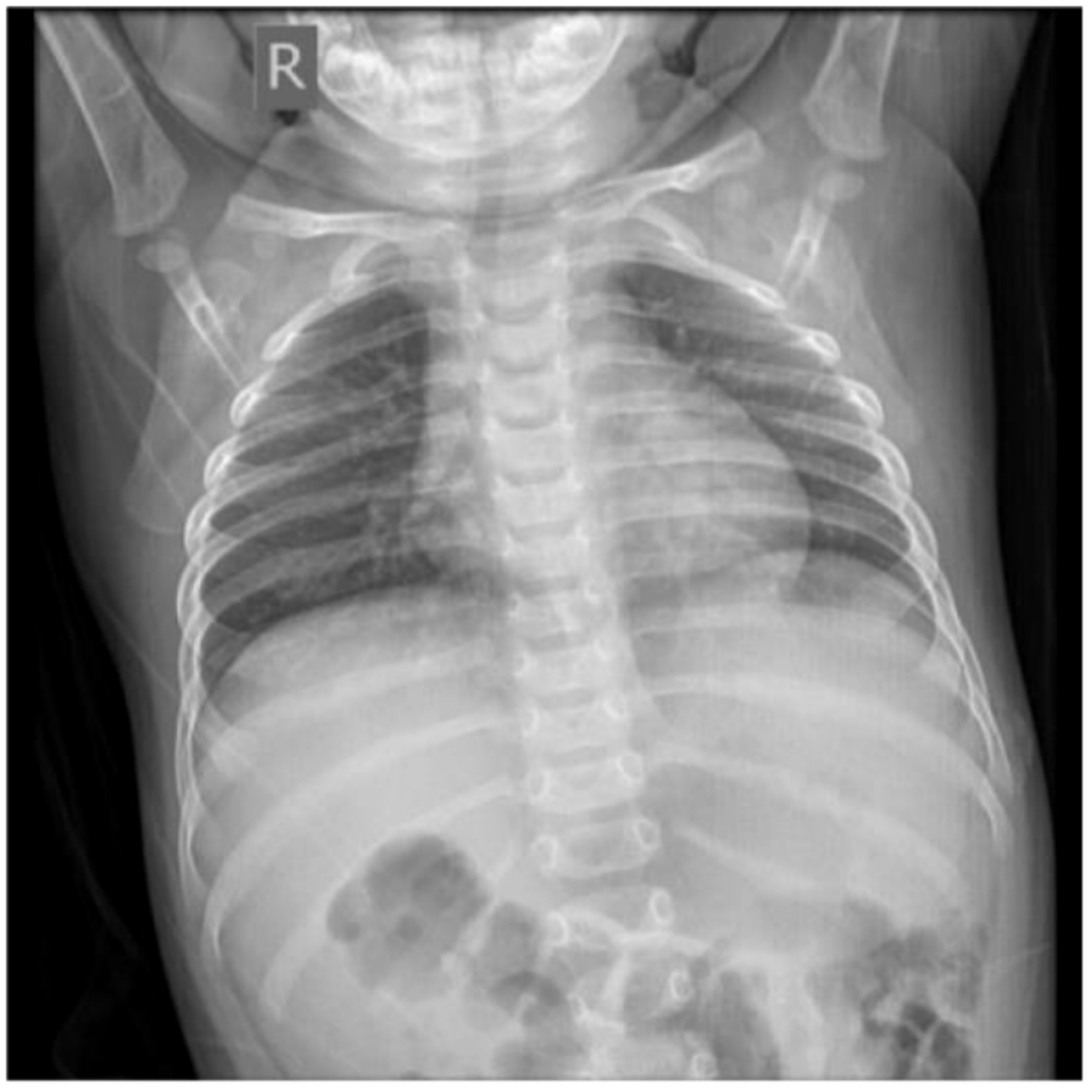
Chest X-ray after recovery.
